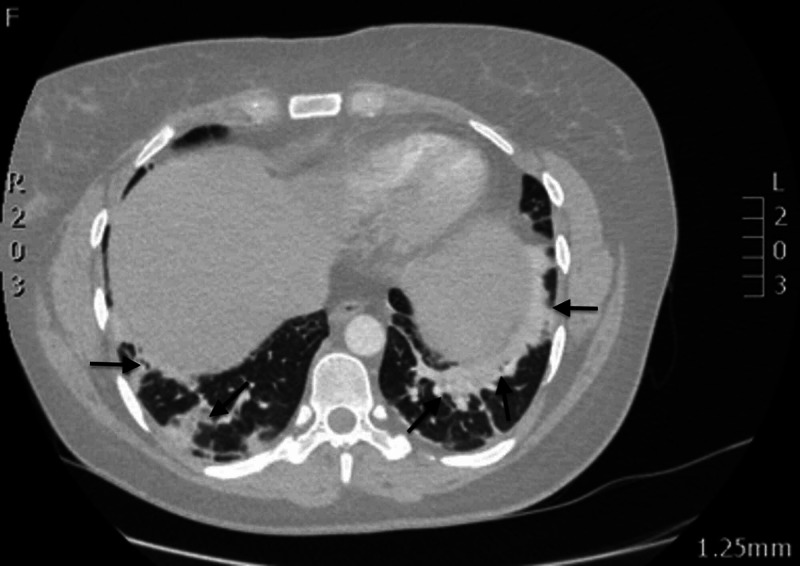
CTPA image (24/09/2020) showing multi-lobar pneumonia in the lower lobes of both lungs (black arrows) CTPA, computed tomography pulmonary angiogram
